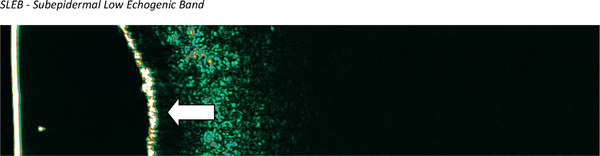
High‐frequency ultrasound image of active discoid lupus erythematosus (facial skin). The arrow indicates SLEB. SLEB, subepidermal low echogenic band.
